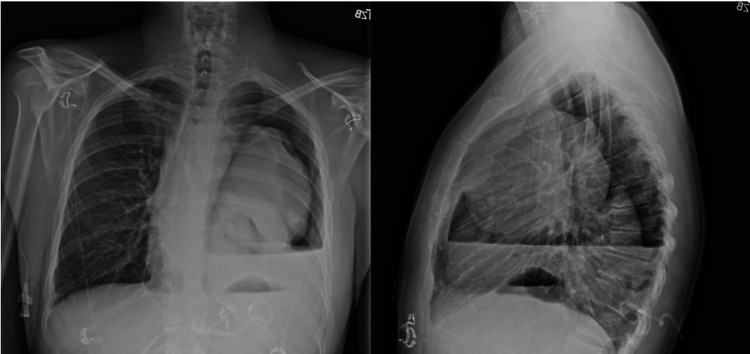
Anteroposterior and lateral chest X-ray images of the chest following drainage of the pleural effusion with persistent left lung atelectasis and large ex vacuo pneumothorax
